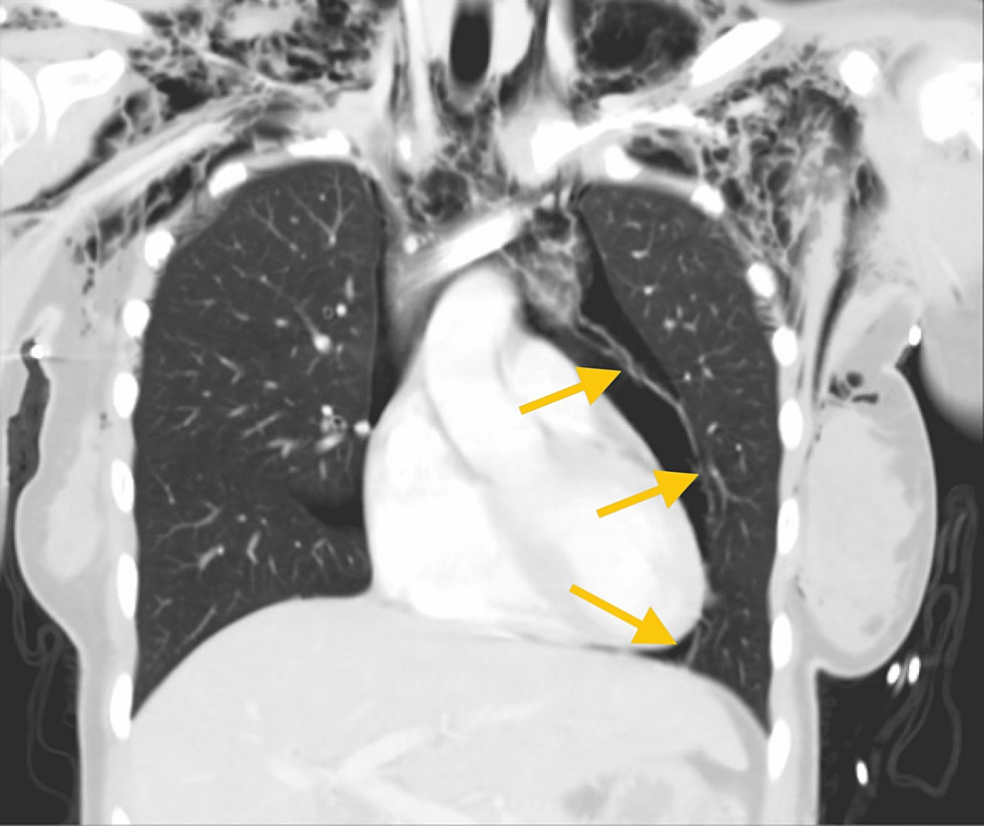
Coronal computed tomography of the chest further demonstrating pneumopericardium (yellow arrows)
